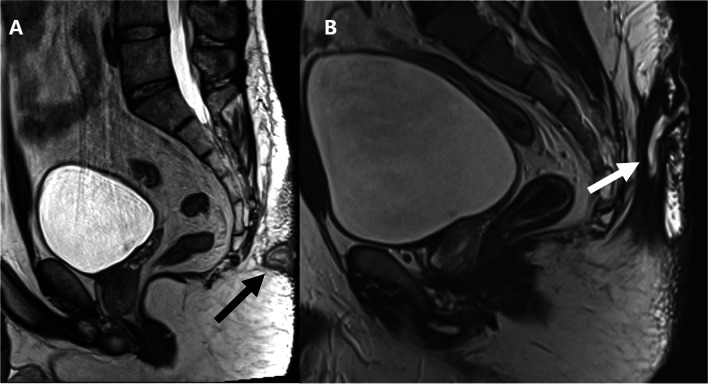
Sagittal T2-weighted images of two different patients with PSD; (A) a 22-year-old male with PSD showing rounded/cystic morphology; (B) a 30-year-old male with tubular branching PSD tract
